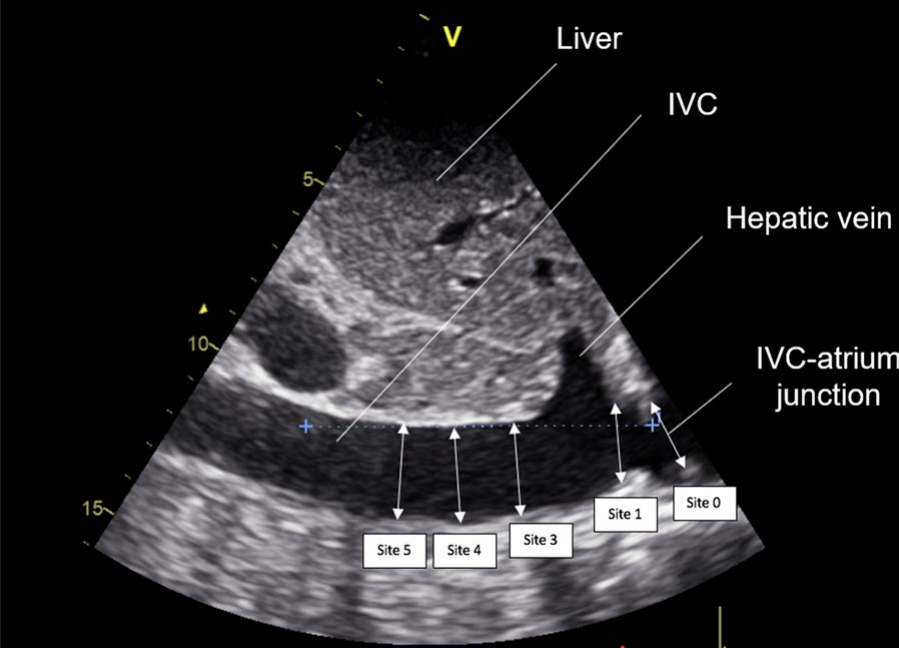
Bi-dimensional ultrasound recording of the inferior vena cava (IVC) generated using the sub-costal, long-axis view. Measurement of IVC diameters were carried out at five sites: at the IVC–right atrium junction (site 0), then at 1 (site 1), 3 (site 3), 4 (site 4) and 5 cm (site 5) caudal to the IVC–atrial junction
